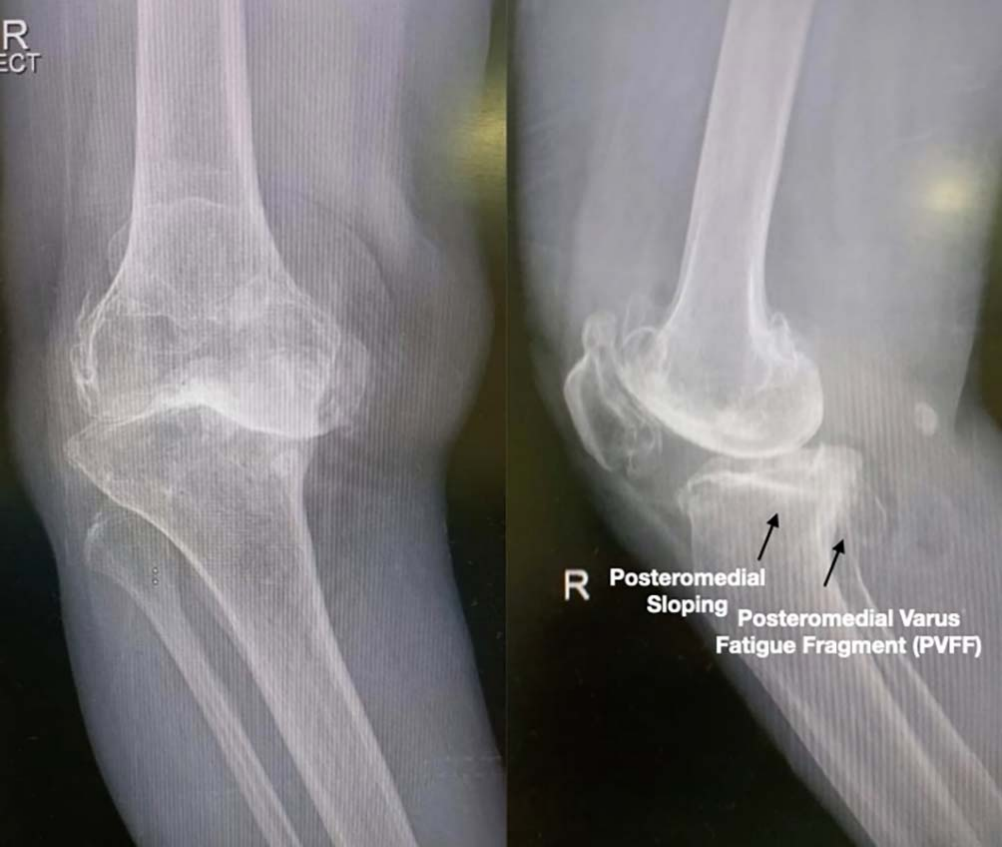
Preoperative radiographs showing varus deformity and posteromedial varus fatigue fragment (PVFF).
